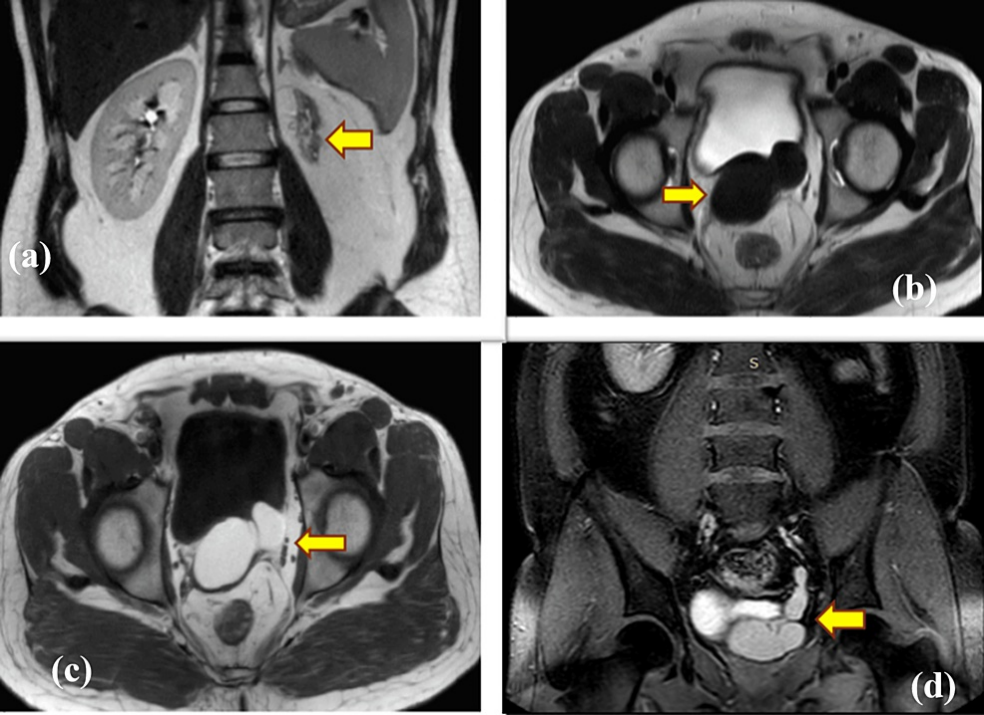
(a) Coronal T2-weighted image shows an atrophic left kidney (yellow arrow), while the right kidney appears normal. (b) The axial T2-weighted image shows a hypointense cystically dilated seminal vesicle, which appears hyperintense on the axial T1-weighted image (c), suggesting a high proteinaceous or hemorrhagic content. (d) The coronary post-contrast T1-weighted image demonstrates the communication of the cystically dilated seminal vesicle with the left ureter, indicating ectopic insertion of the ureter into the seminal vesicle.
